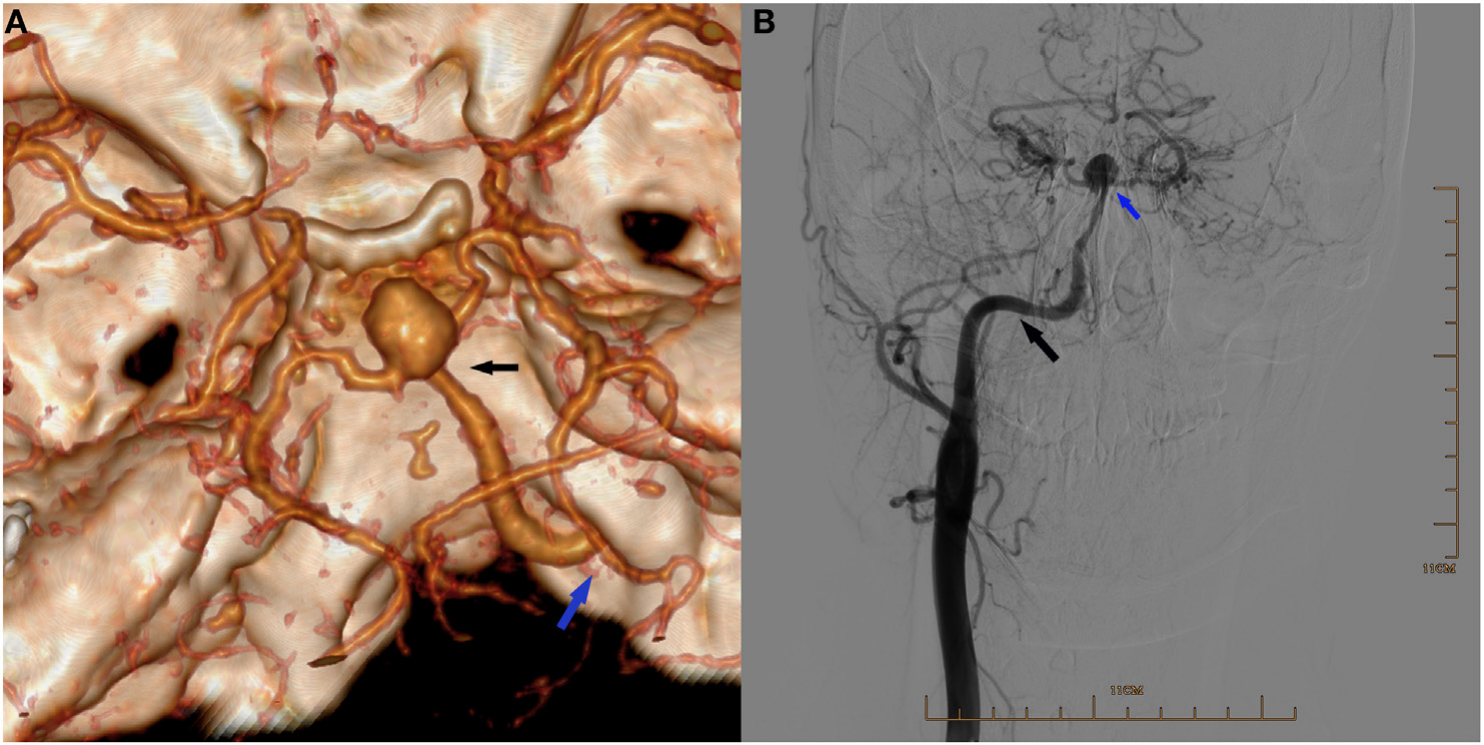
(A) Three-dimensional reconstruction of computed tomography angiography showing an aneurysm located at the bifurcation of the right persistent primitive hypoglossal artery (PPHA) and two posterior cerebral arteries (black arrow), and the right PPHA entering the skull *via* the right hypoglossal canal (blue arrow) and supplying the circle of Willis. (B) Digital subtraction arteriogram of the right common carotid artery (CCA) showing the PPHA (black arrow) originating from the CCA and an aneurysm in the apex (blue arrow). The right internal carotid artery was absent.
